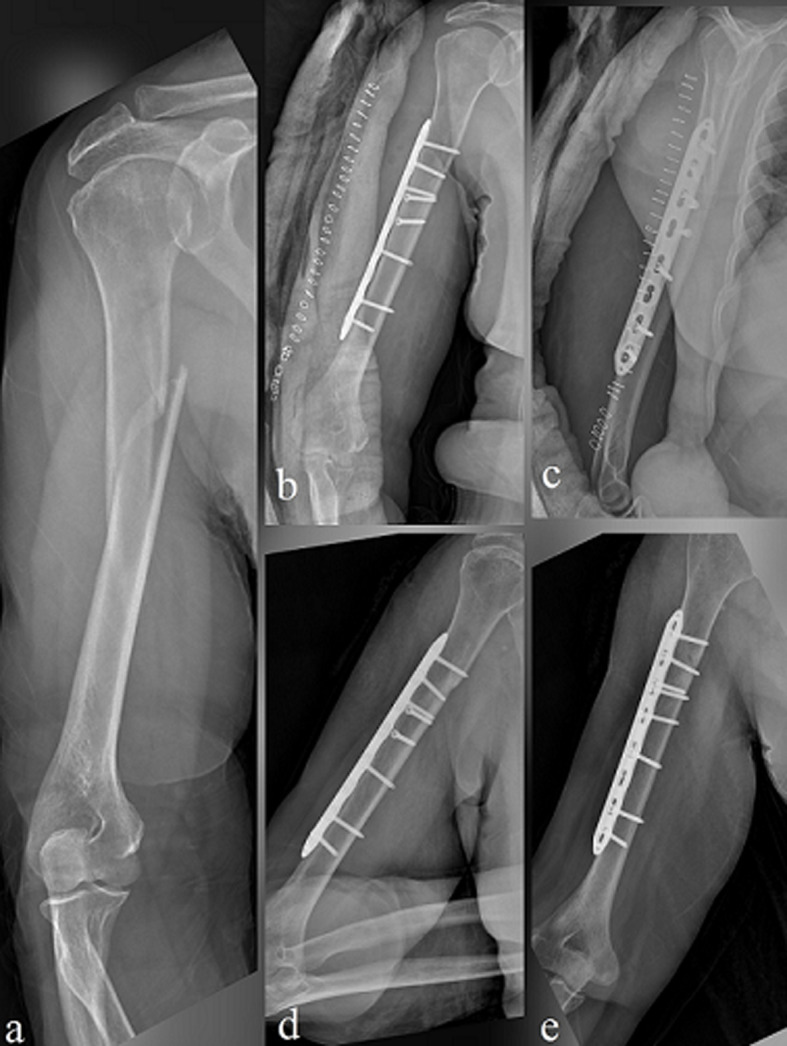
4.5 mm single LCP fixation; X-ray images of a 29-year-old male patient with a right humeral shaft fracture as a result of a sports injury: (a) first anteroposterior (AP) plane X-ray image; (b and c) early postoperative X-ray images; (d and e) X-ray images at the 6^th^-month postoperative control
